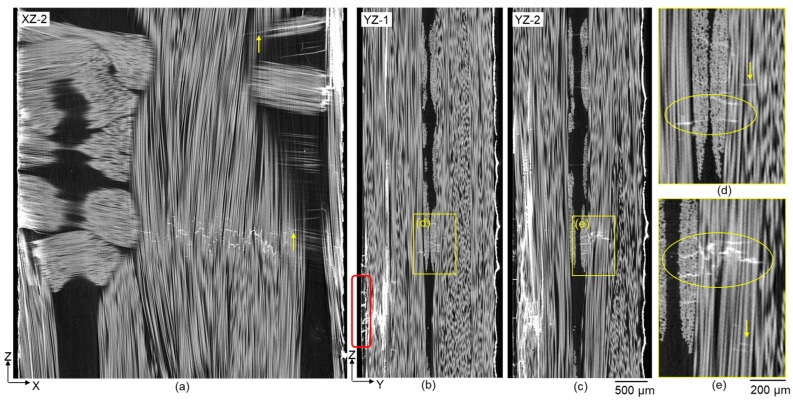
X-ray CT virtual (a) XZ-2 and (b) YZ-1 and (c) YZ-2 sections (positions indicated in Figure 3b,c) recorded after 500,000 cycles showing crack paths connecting off-axis cracks in the backing fibre bundles and fibre fractures in the UD fibre bundles. (d,e) Magnfied views of the highlighted regions in (b,c) showing the connected off-axis cracks (marked with ellipses) and also isolated UD fibre fractures (marked with downward-arrows).
